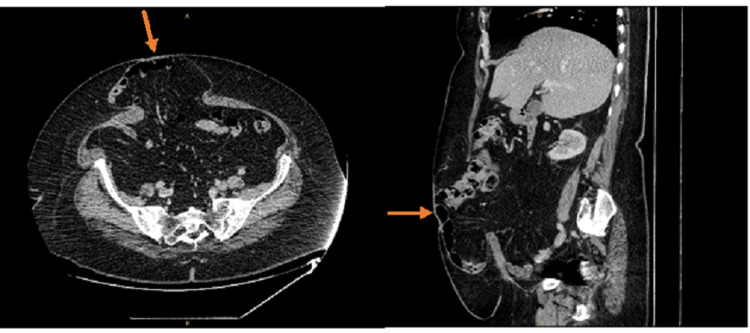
Pre-op CT abdomen prior to incisional hernia repair. Axial view on left, midline sagittal view on the right. Orange arrows indicate hernia.
